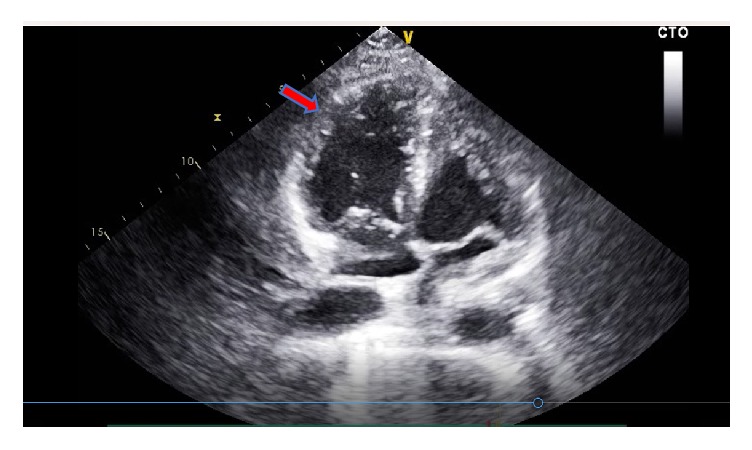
Transthoracic echocardiogram obtained before administration of t-PA: apical views showing a severely dilated right ventricle (RV) with reduced right ventricular systolic function. RV free wall is hypokinetic and RV apical wall is hypercontractile (arrow; McConnell sign). Left ventricular size and systolic function are normal.
